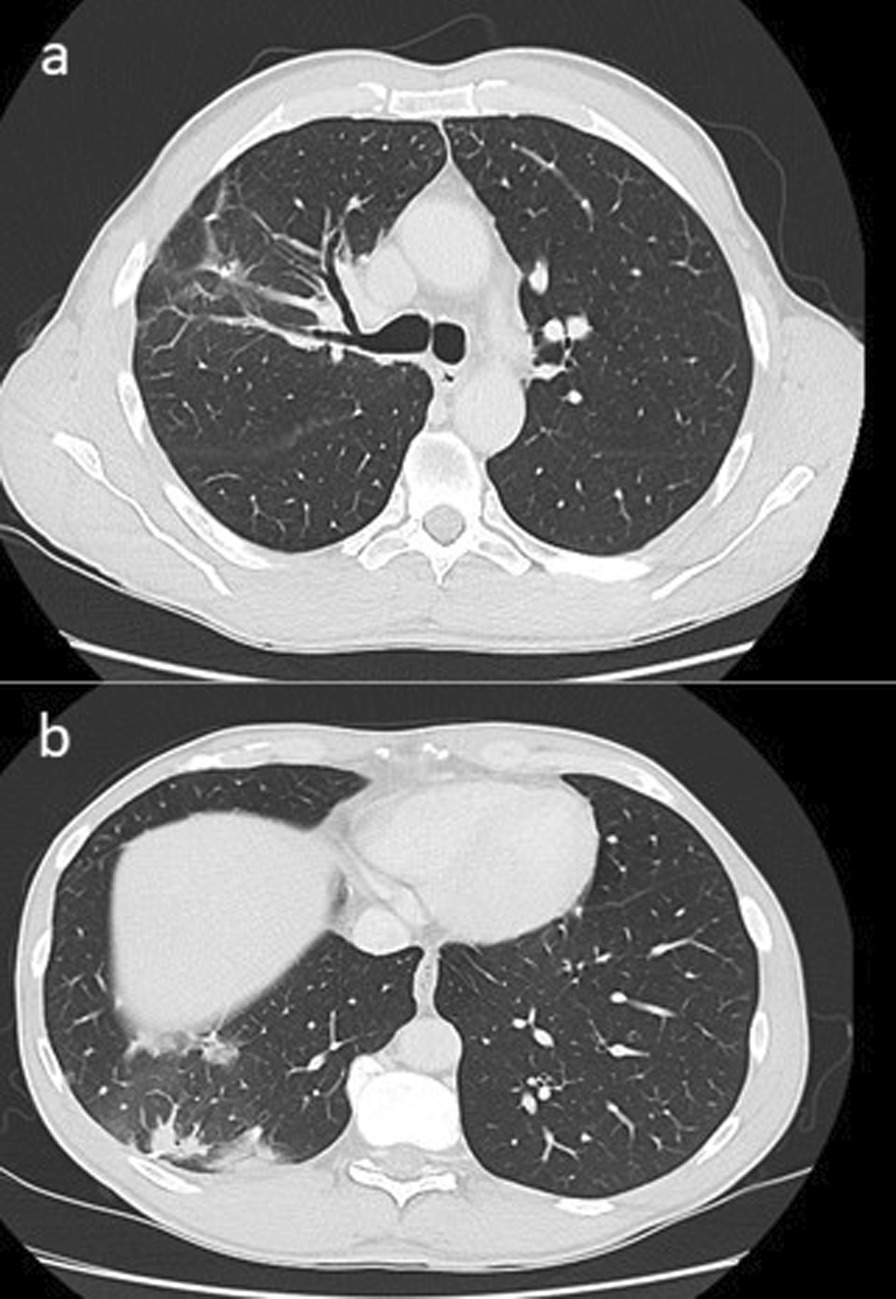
Axial slices of computed tomography chest 6 months post-SABR. Post-surgical and radiotherapy changes in the right lung with no evidence of progression. a Right upper lobe. b Right lower lobe
